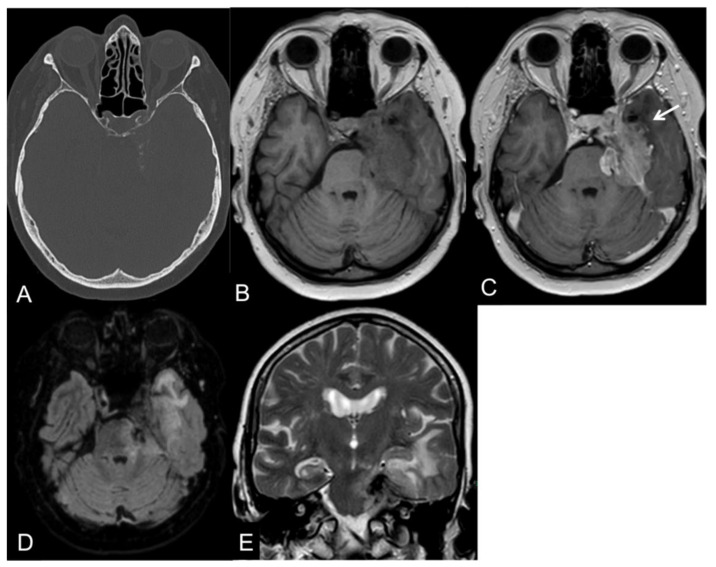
CT and MR images of a 69-year-old female patient with an anaplastic petroclival meningioma (III). The tumor shows calcifications in the CT scan (A). On T1-weight images, the intratumoral signal is iso- to hypointense in comparison to the gray matter (B) with a vivid contrast enhancement after intravenous gadolinium-based contrast enhancement (C) that extends into the adjacent brain parenchyma, representing parenchymal infiltration (arrow). On fluid-attenuated inversion recovery (FLAIR; (D)) and T2-weighted images (E), the tumor presents a central low signal intensity representing the intratumoral calcifications, an absence of the CSF cleft, and a moderate perifocal edema.
